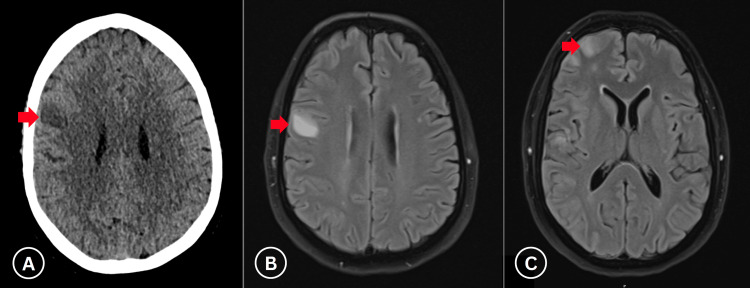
Initial imaging following symptom onset. (A) Non-contrast CT shows right frontal and peri-rolandic hypodensity consistent with acute middle cerebral artery territory infarction. (B, C) MRI demonstrates multiple enhancing cortical lesions in the right hemisphere (red arrows), initially suspicious for metastatic disease.
